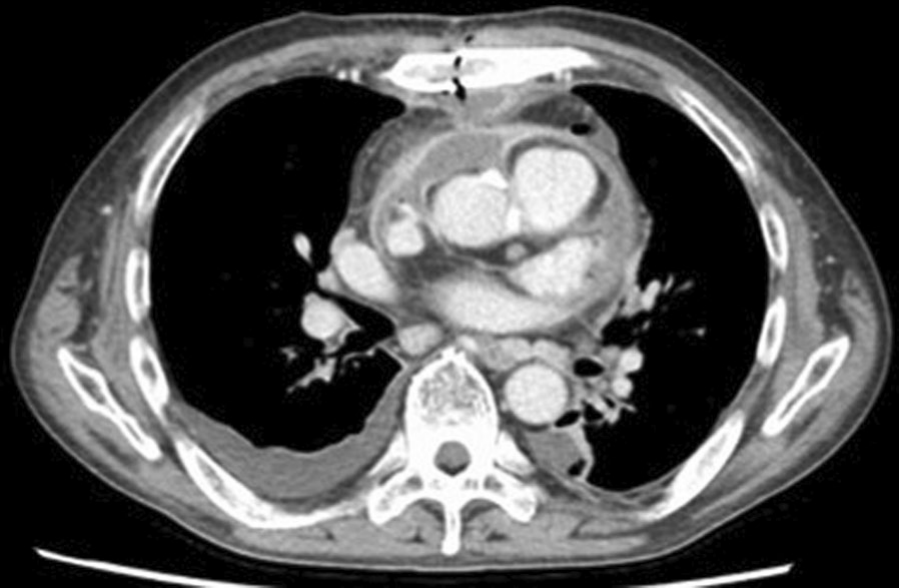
Computed tomography showing retrosternal fluid and free air, indicating mediastinitis
